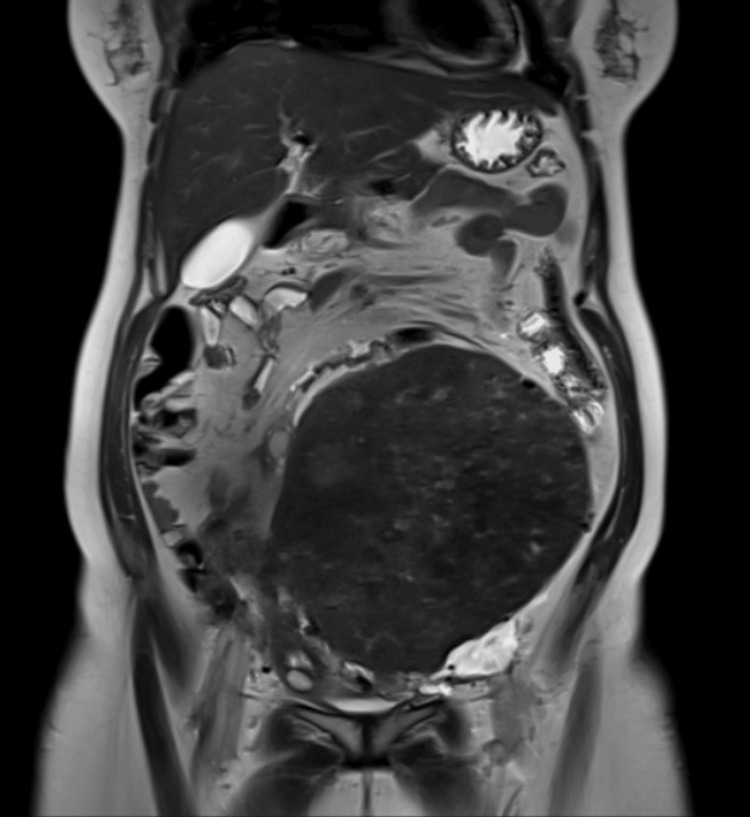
Case 5: MRI of the abdomen and pelvis, axial T2-weighted sequence Axial T2-weighted MRI demonstrating the large, well-defined ovoid mass measuring 21 × 13 × 17 cm in the left adnexal region, occupying the pelvis and displacing the uterus and bowel superiorly and to the right. The mass demonstrates heterogeneous signal intensity with peripheral cystic areas, consistent with the ovarian pedicle sign identified on imaging.
